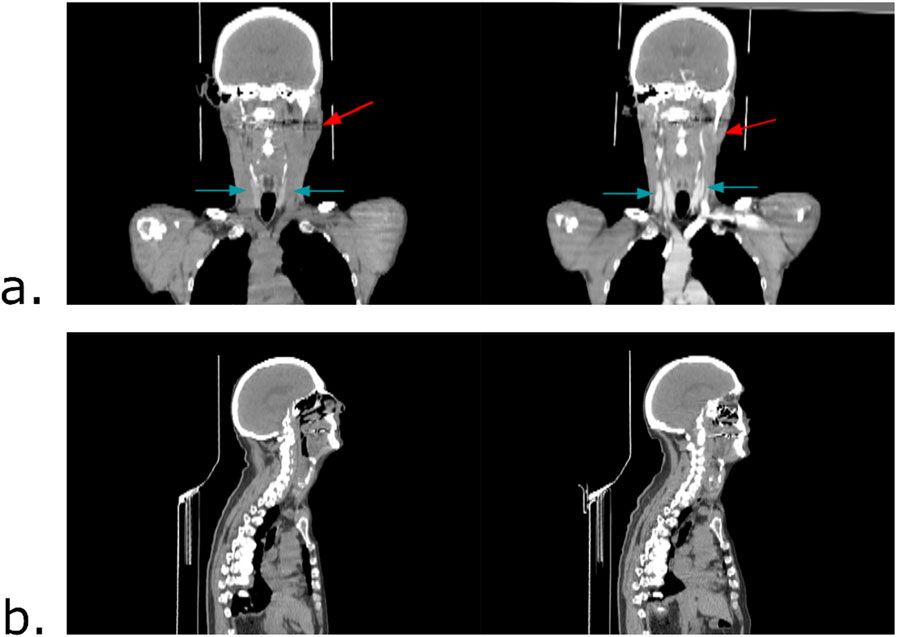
Intra-individual differences that can be found in CT images acquired at different time points. Left: pre-treatment CT. Right: post-treatment CT. a Red arrow: changes of patient anatomy after the treatment. Blue arrow: intensity differences due to the injection of a contrast agent in the post-treatment CT (right). b Differences in the position of the patient between pre- and post-treatment CT. Here, the inclination of the head has changed
